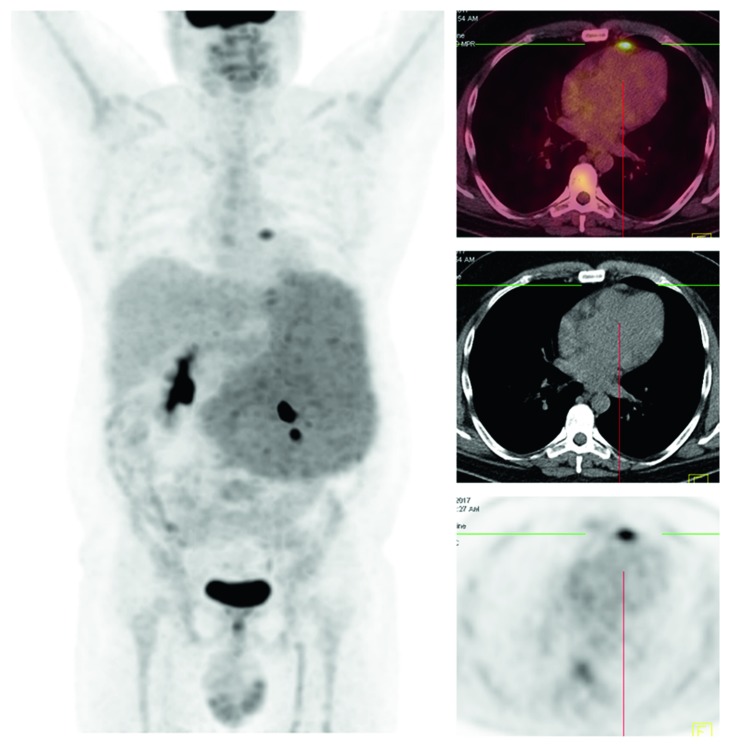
Positron emission tomography-computed tomography. Enlarged pericardial lymph node in the anterior mediastinum with SUVmax = 6.1.
